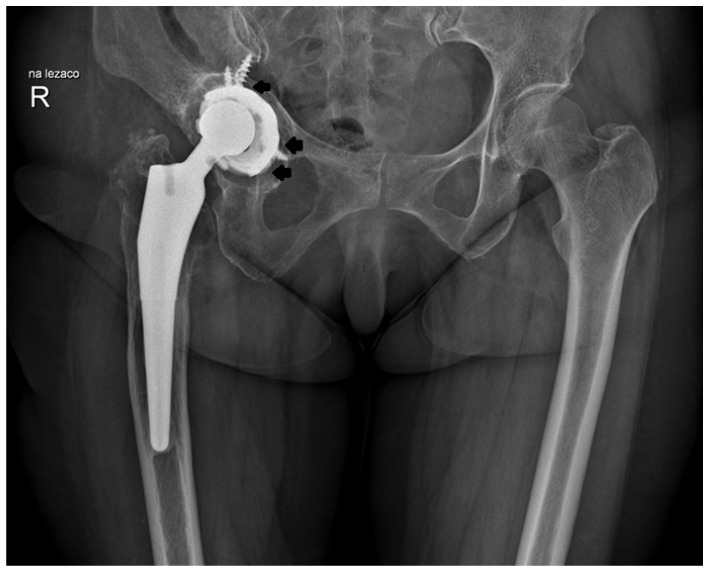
X-ray images of patient who qualified for right hip revision surgery. The arrows indicate the loosening of the acetabular element.
